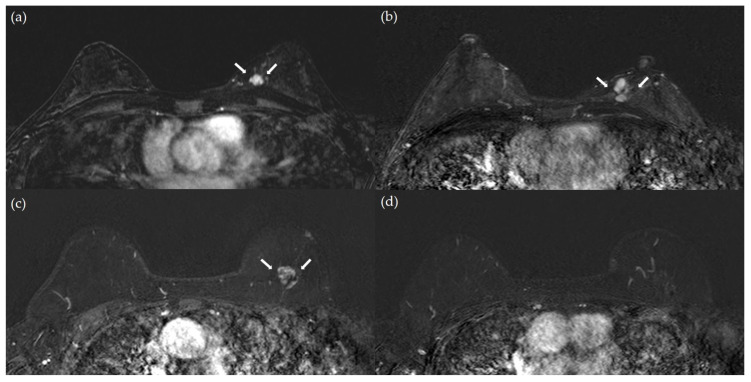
Representative cases of a subsequent breast cancer event and a non-subsequent breast cancer event: (a,b) A 28-year-old woman with ER/PR-positive, HER2-negative breast cancer and high nuclear grade. (a) Preoperative contrast-enhanced T1-weighted MR image with subtraction shows a 1.5 cm irregular, homogeneously enhancing mass in the upper medial quadrant of the left breast (arrows). (b) Postoperative contrast-enhanced T1-weighted MR image with subtraction obtained 60 months later reveals a new irregular mass in the medial central portion of the left breast (arrows), confirmed as a subsequent breast cancer event by core needle biopsy. (c,d) A 58-year-old woman with ER/PR-positive, HER2-negative breast cancer without high nuclear grade. (c) Preoperative T1-weighted MR image with subtraction shows a 2.0 cm irregular mass in the upper medial quadrant of the left breast with heterogeneous internal enhancement, characterized by nonuniform signal intensity and intermixed enhancing and nonenhancing areas (arrows). (d) Postoperative contrast-enhanced T1-weighted MR image obtained 60 months later shows no evidence of subsequent breast cancer event.
